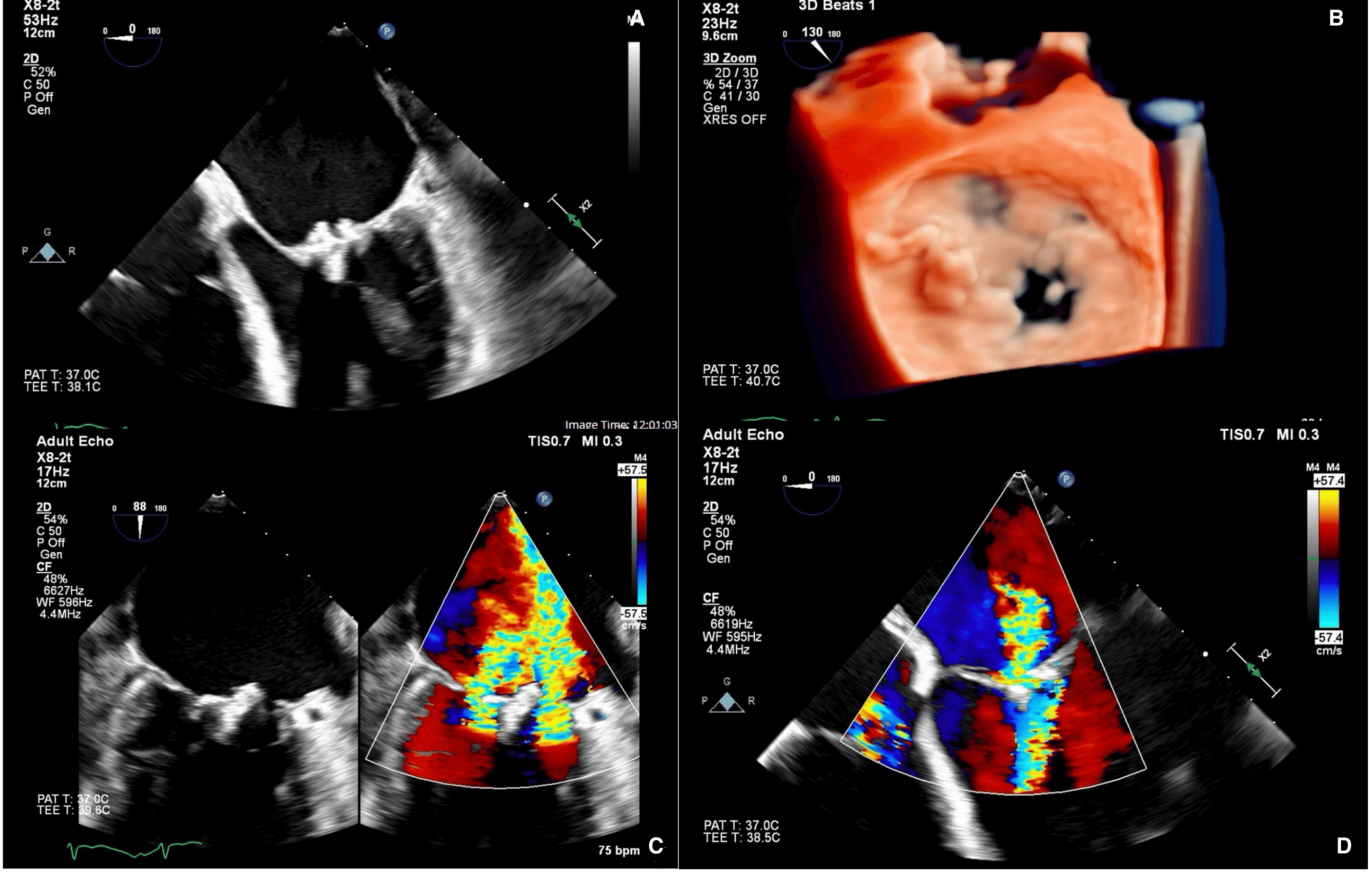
Preoperative transesophageal echocardiogram showing mitral valve vegetation and regurgitation. A: 2-dimensional view of mitral valve vegetation; B: 3-dimensional view of mitral valve vegetation; C, D: color Doppler view showing mitral valve regurgitation
